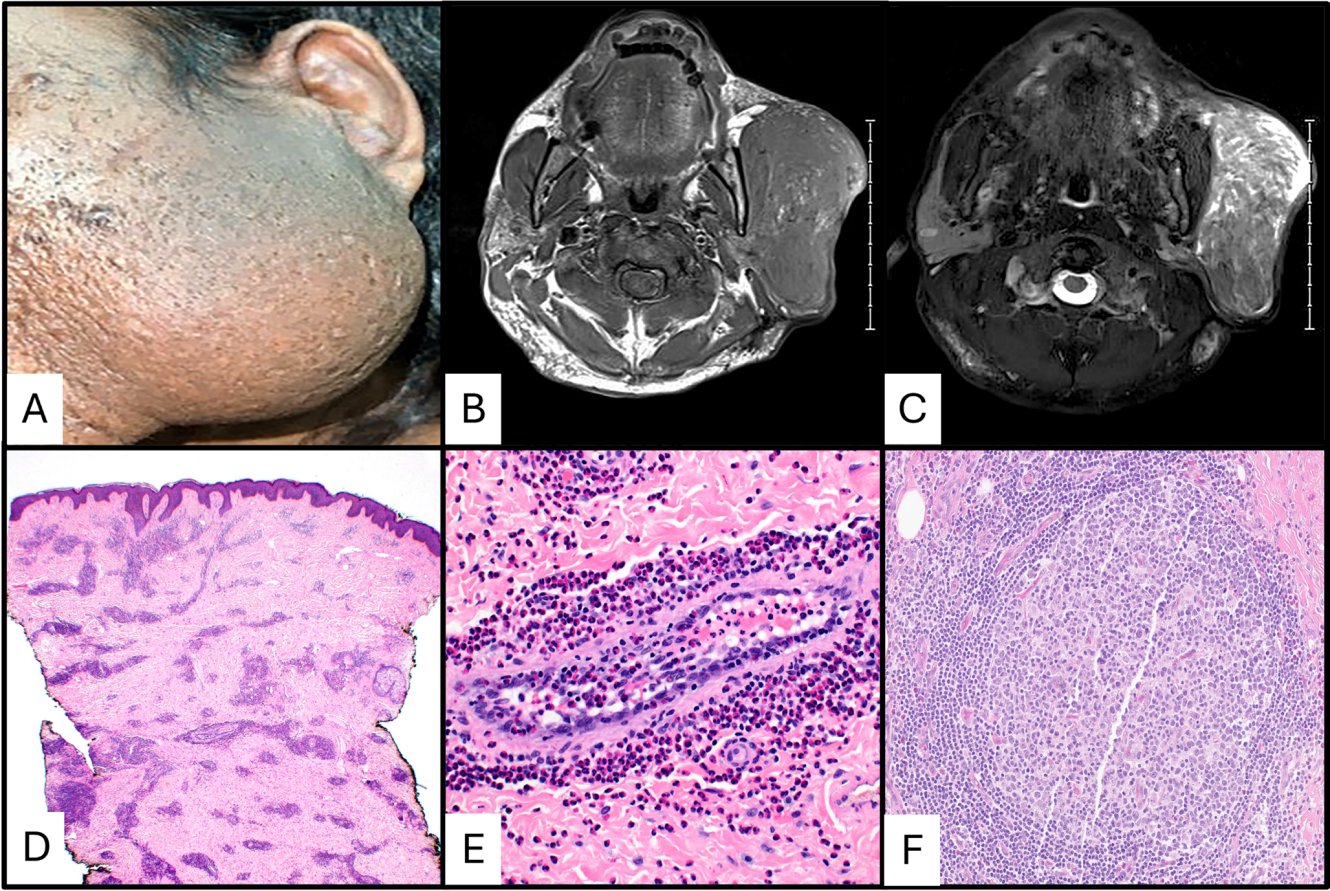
41-year-old male (Marshal Islander/Asian/Pacific Islander) presented with a large, recurrent parotid mass eight years after initial surgical excision (a). The patient’s serum IgE level was 5783 IU/ml (normal range 6-495 IU). Magnetic resonance imaging revealed a large T1 isointense mass in the left parotid region (b). The mass is hyperintense on the T2-weighted image (c). An additional lesional focus is present in the postauricular region. The mass on punch biopsy (d) showed a deeply seated lesion composed of a mixed inflammatory infiltrate with prominent eosinophilia and indistinct margins. Eosinophilic vasculitis (e) and subcutaneous secondary follicles with surrounding eosinophils (f) were present
